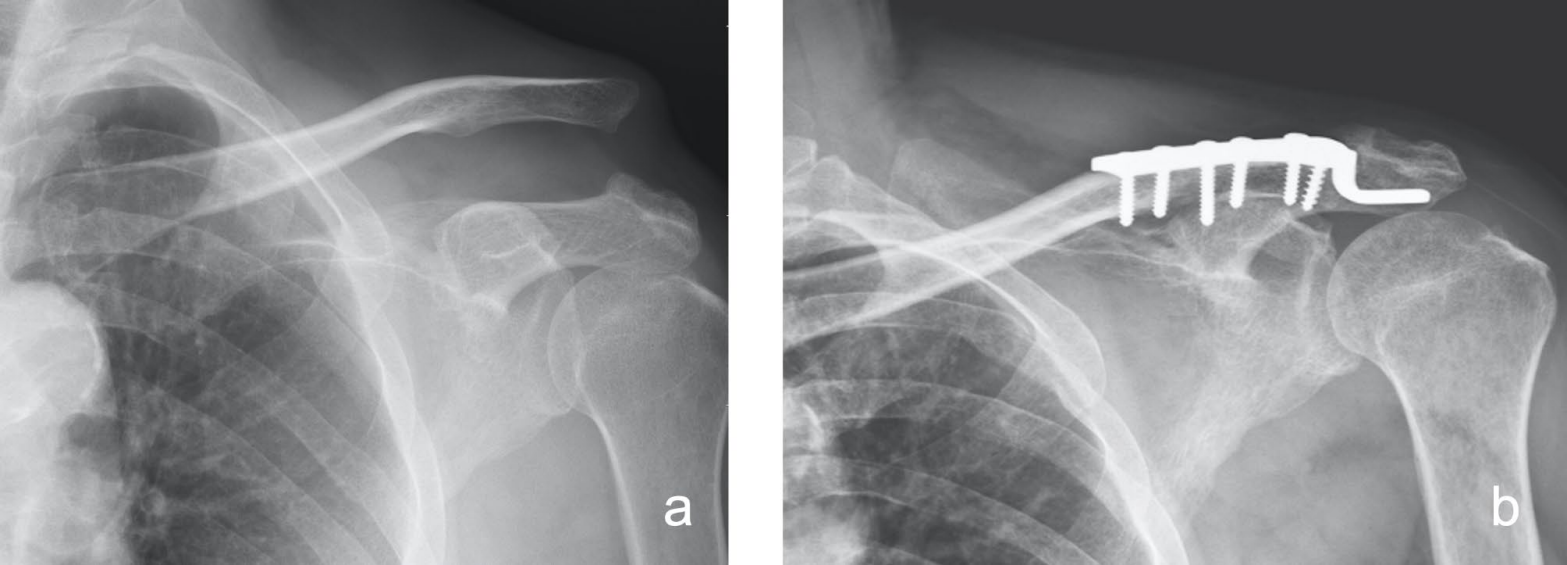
The hook plate fixation modality for a type V AC joint dislocation. (a) Preoperative plain radiograph. (b) Postoperative 1-month plain radiograph
